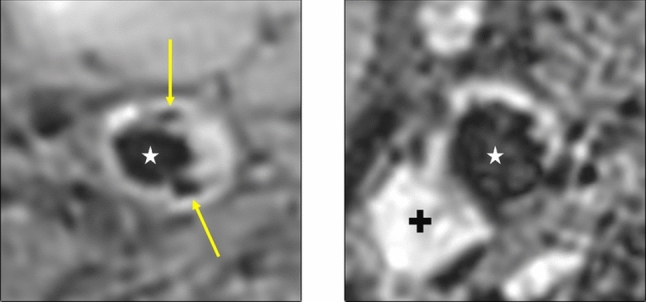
T1-weighted BB-MRI of two carotid arteries. The lumen is marked with a white star. On the left, calcification is present. The calcification has low signal intensity. Calcified areas are marked with a yellow arrow. On the right, intraplaque hemorrhage was detected. Hemorrhage has high signal intensity in T1-weighted BB-MRI and is marked with a black plus
